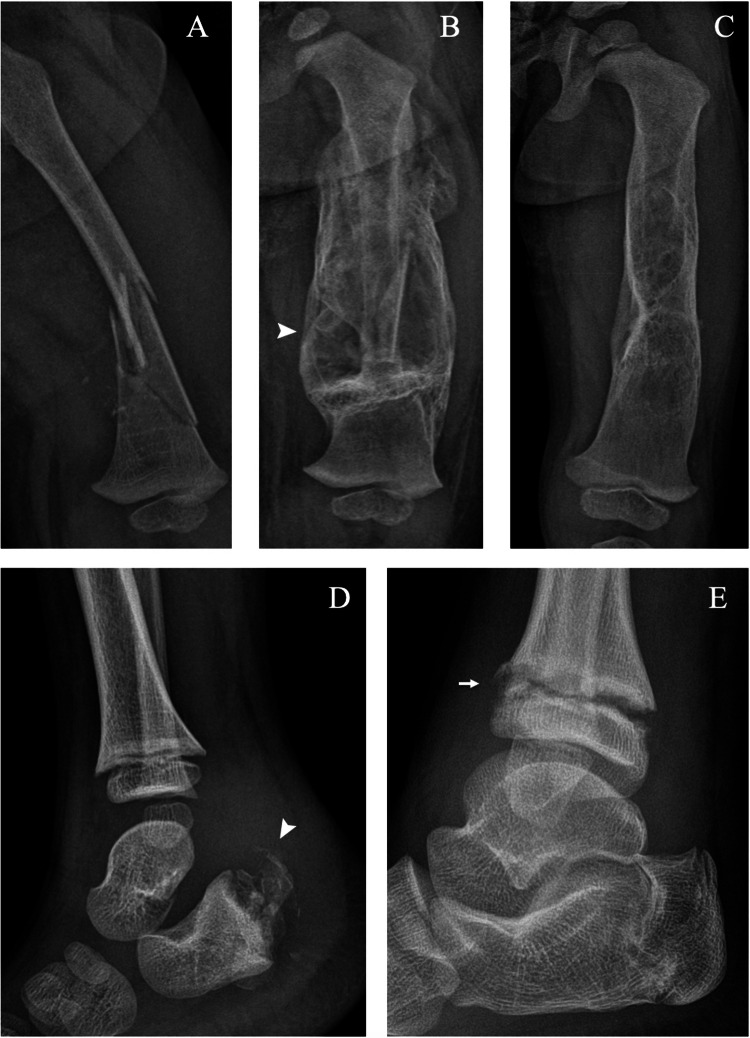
Two cases of lower-limb fractures of the left femur of a 1-year-old boy with congenital insensitivity to pain (A–C) and the right calcaneus of a 5-year-old boy with hereditary sensory and autonomic neuropathy type IV (D, E). Anteroposterior radiographs of the femoral fracture at injury (A), 3 months (B) and 13 months (C) show a large subperiosteal haematoma (arrowhead) (B) and the ongoing remodelling of the femoral diaphysis after complete healing (C). Lateral radiographs of the calcaneal fracture (arrowhead) at injury (D) and 10 years later (E) show complete healing without deformity; note the epiphyseal separation (arrow) of the tibia
